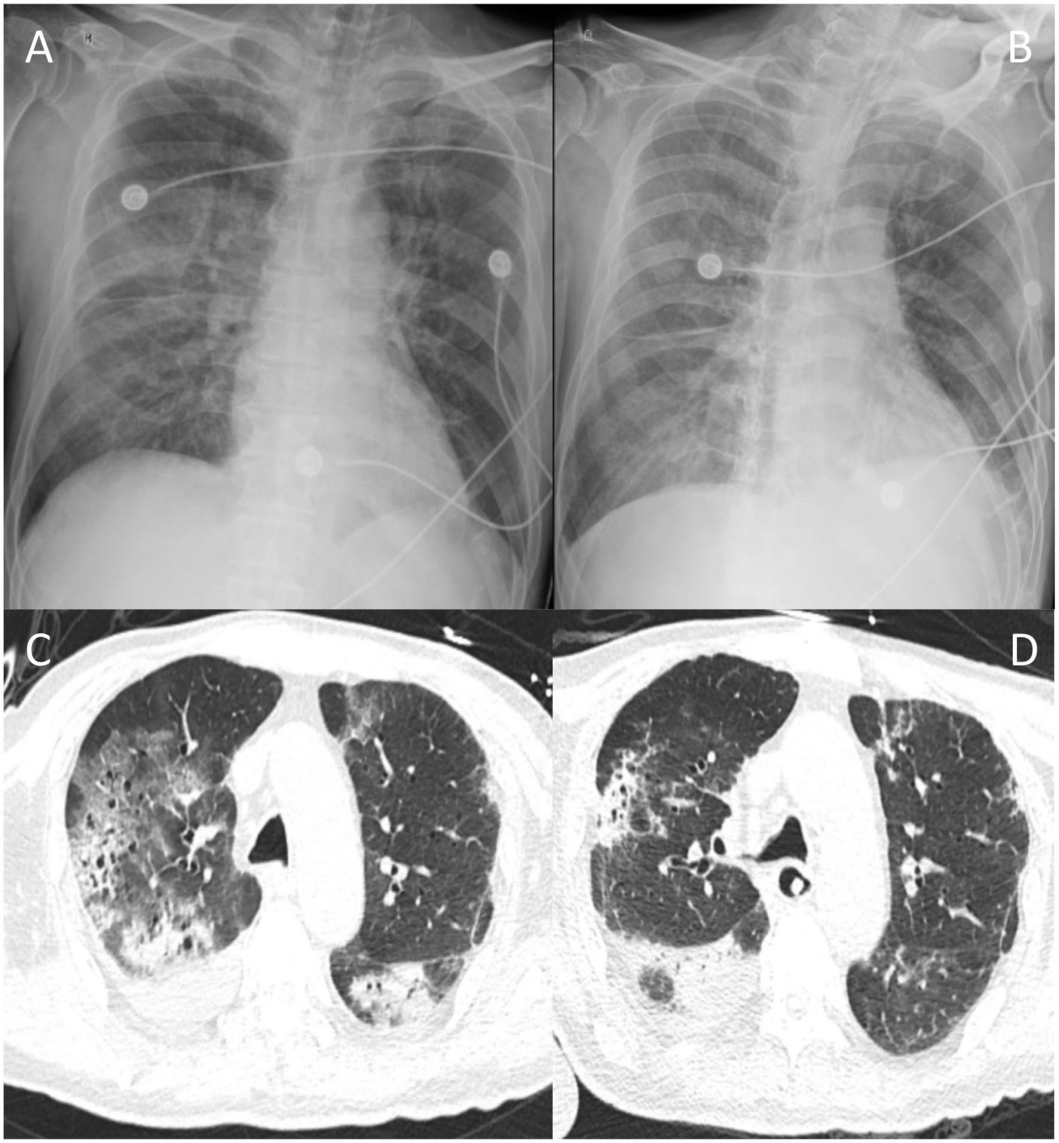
Typical chest radiographs of a patient with COVID-19. Chest radiographs of one patient on day 1 at ICU admission (A) and day 5 (B); CT scan of chest of the same patient on day 10 (C) and day 20 (D) after ICU admission.
